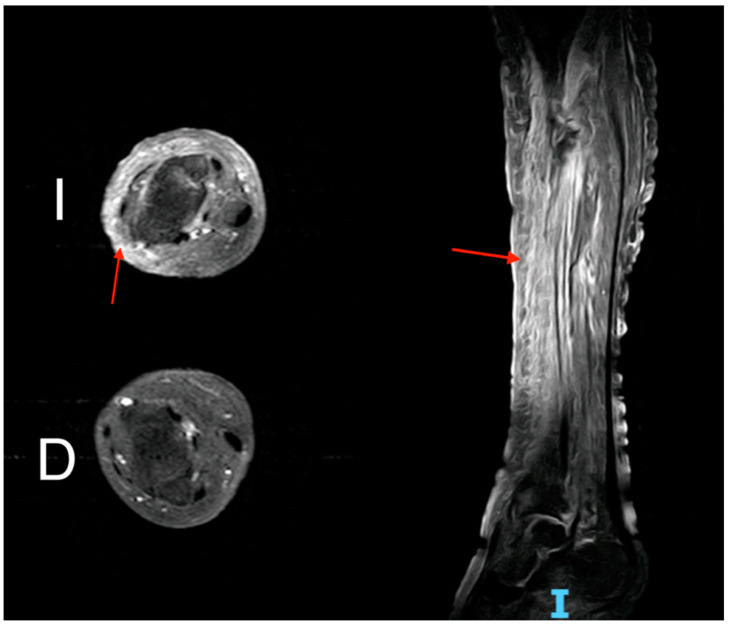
Magnetic resonance imaging of legs in axial (left) and sagittal (right) views. Arrows show the deep involvement of the infection with hyperintense image.
